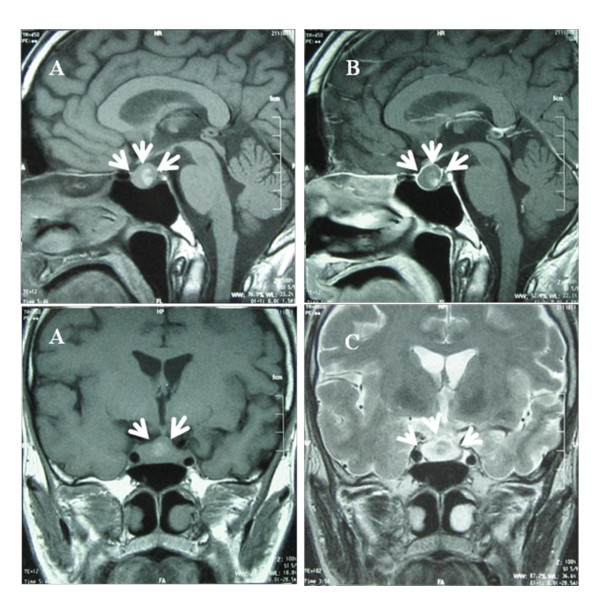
MRI scan demonstrating a clearly defined intrasellar mass. (A) and (B) Preoperative sagittal T1-weighted MRI (T1WI) showing a suprasellar round tumor measuring 15 × 20 mm in diameter (arrows) (A) and heterogeneous enhancement by gadolinium (Gd) contrast medium (T1WI+Gd) (arrows) (B). (C) Coronal T2-weighted MRI (T2WI) showing the tumor as partially hypointense (arrows).
